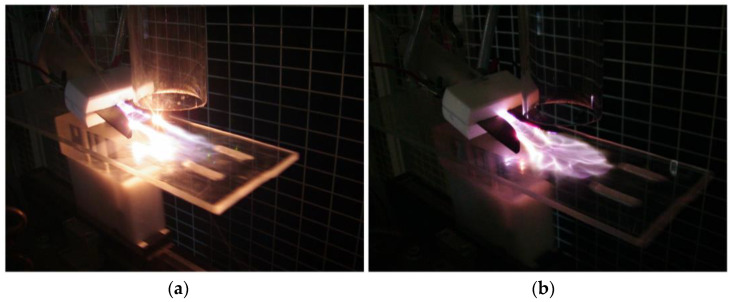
Photo images of the gliding arc (a) without ultrasonic irradiation, and (b) with ultrasonic irradiation. Reproduced with permission from [109] Kusano et al., *Surf. Eng.*; published by Taylor & Francis, 2012.
